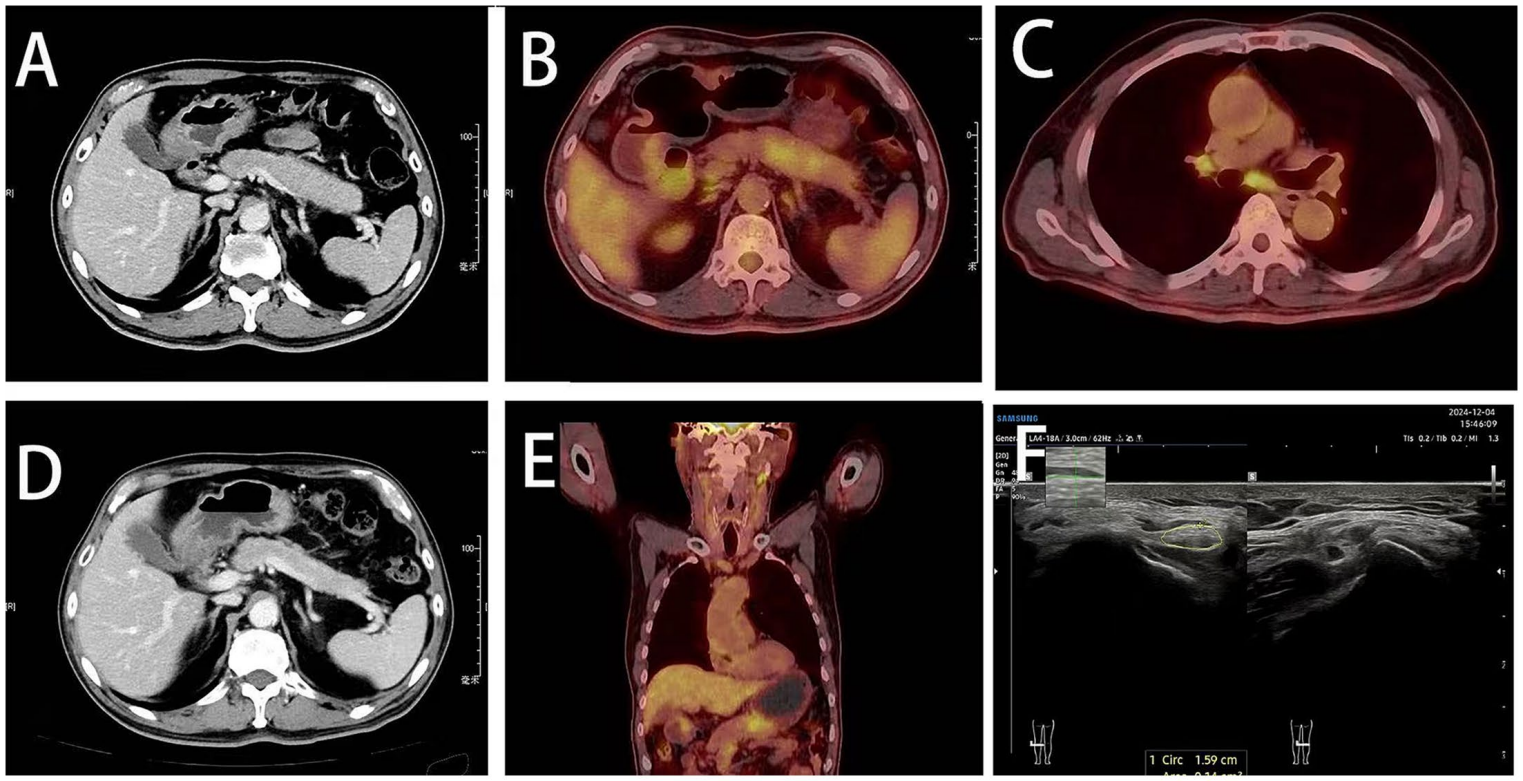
Radiographic features. (A) Enlarged body and tail of the pancreas visualized on abdominal CT (pre-steroid therapy). (B) PET-CT showed enlarged body and tail of the pancreas. (C) Enlarged lymph nodes in mediastinal zones 4R and 7 on PET-CT (pre-steroid therapy). (D) Abdominal CT showed pancreas with decreased swelling after treatment. (E) PET-CT showed swollen lymph nodes in the neck (pre-steroid therapy). (F) Lower limb nerve ultrasound reveals several nerve bundles of the right common peroneal nerve are enlarged (before steroid use).
